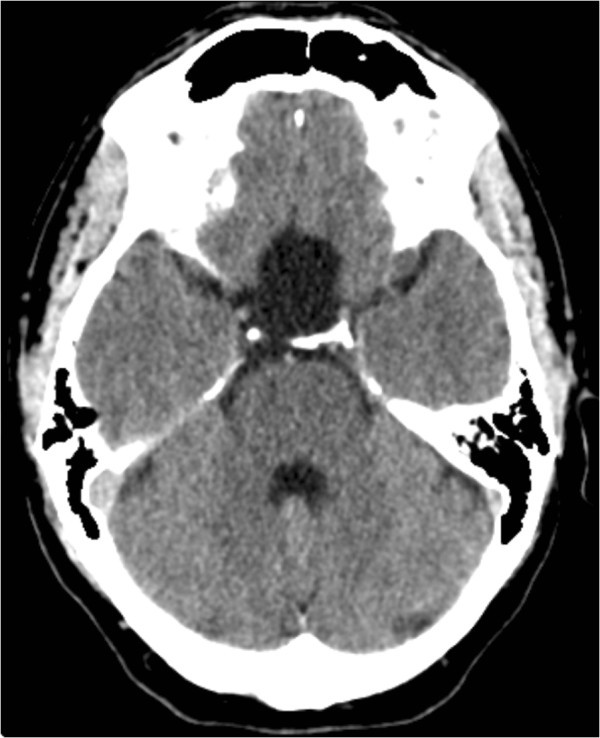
CT of the brain demonstrating a large supra- and intrasellar arachnoid cyst with mass effect on adjacent structures.
